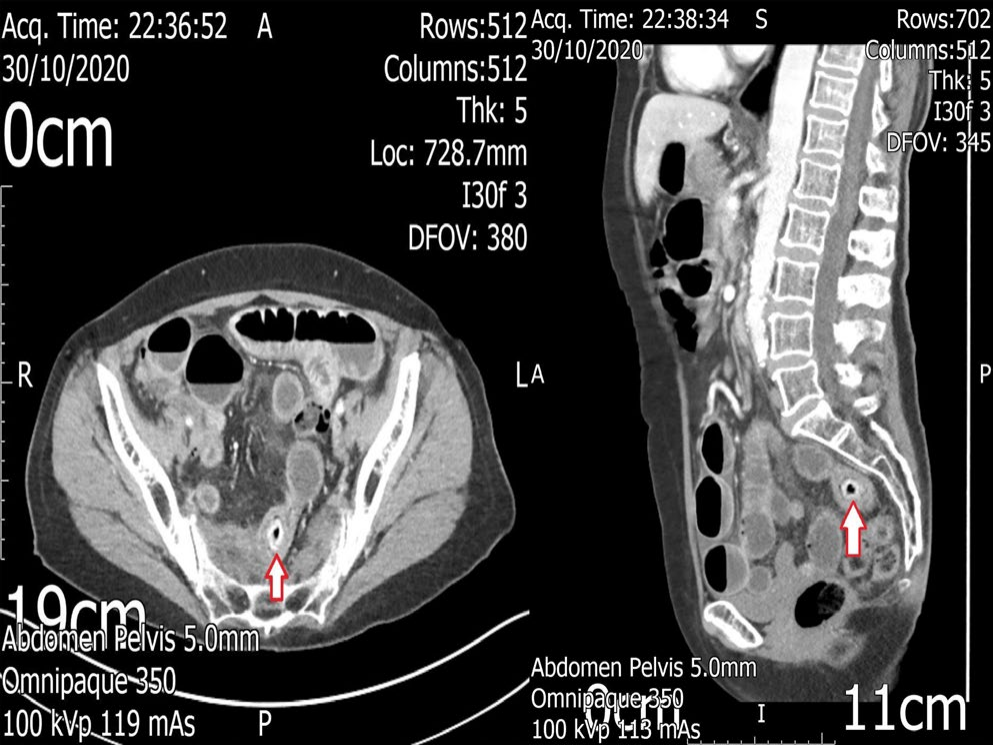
Axial and sagittal CT images of the patient, with evident small bowel obstruction secondary to the impacted enterolith within a thickened ileal loop (red arrows)
